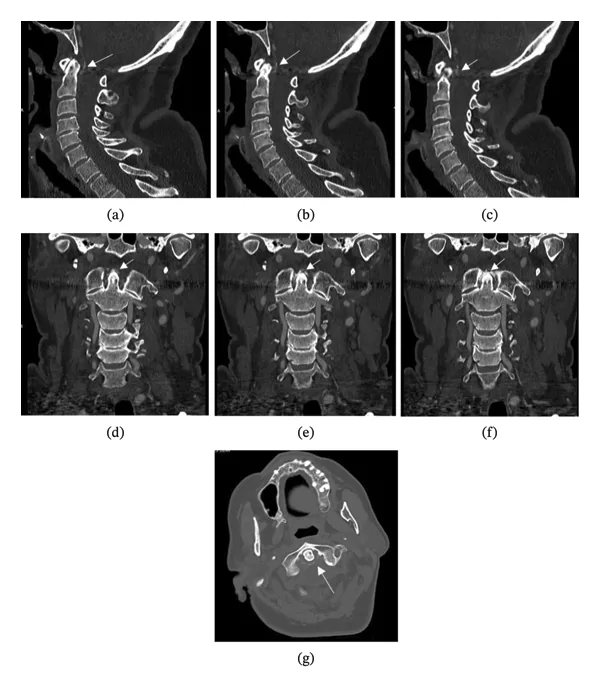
CT demonstrating calcification along the transverse ligament of the odontoid process. (a–c) Sagittal view. (d–f) Coronal view. (g) Axial view.
